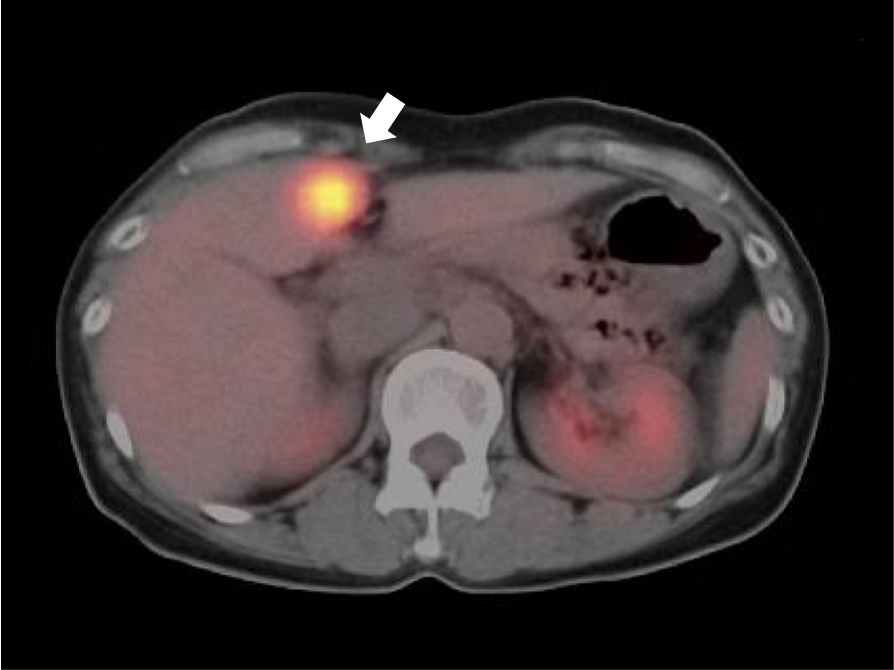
Abdominal computed tomography. Postoperative abdominal computed tomography revealed an ill-defined mass (*white arrow*), 16 × 18 mm in diameter, on liver segment IV
